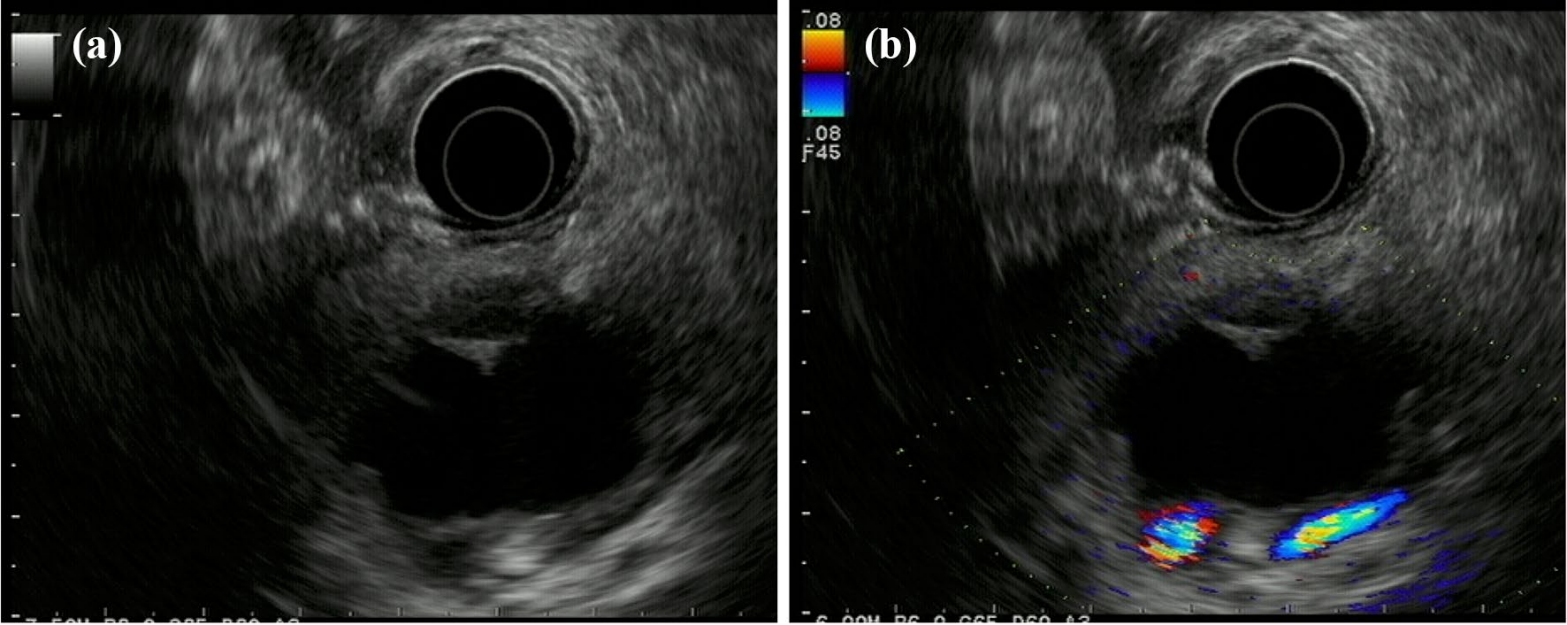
Endoscopic ultrasound showed a cyst-in-cyst structure (a, b)
